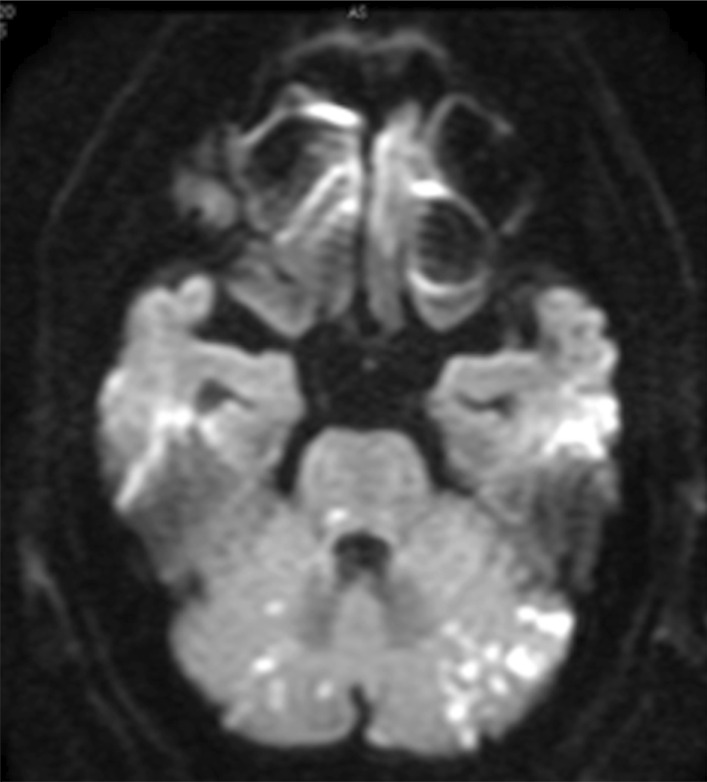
Magnetic resonance imaging showing multiple high-intensity areas in the brain, mainly cerebellum
